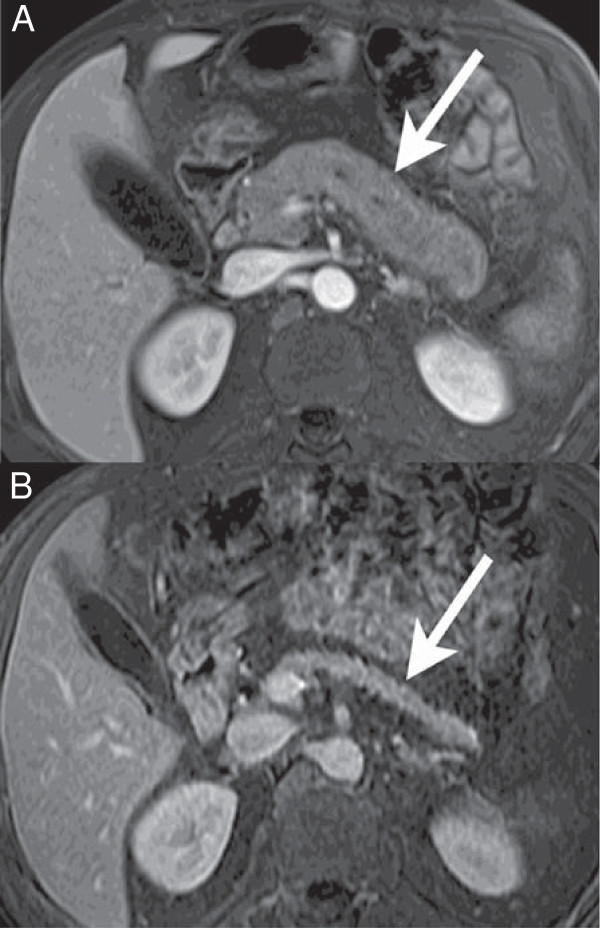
Post-therapeutic pancreatic atrophy. A. Axial contrast-enhanced T1 weighted MR image through the pancreas depicts an enlarged organ (arrow) with poor enhancement. Irregular beading of the pancreatic duct can be seen even on this image. B. Follow-up scan at the same level 12 months post steroid therapy shows marked atrophy of the pancreas (arrow) with normal enhancement.
